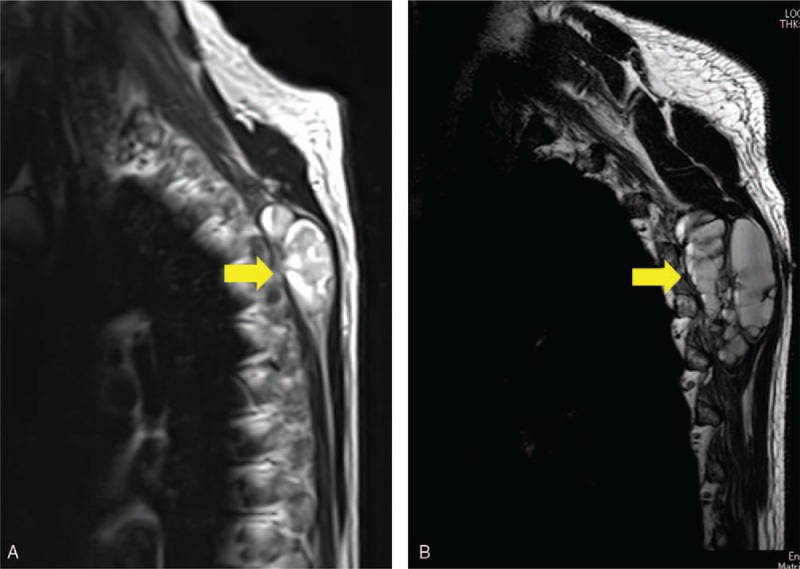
MRI. (A) MRI showing a tumorous mass of 54 × 43 × 30 mm in the latissimus muscle of the back (arrow). (B) After 2 cycles of GD therapy, MRI showed that the size of the back mass was increasing (arrow). GD = gemcitabine+docetaxel, MRI = magnetic resonance imaging.
